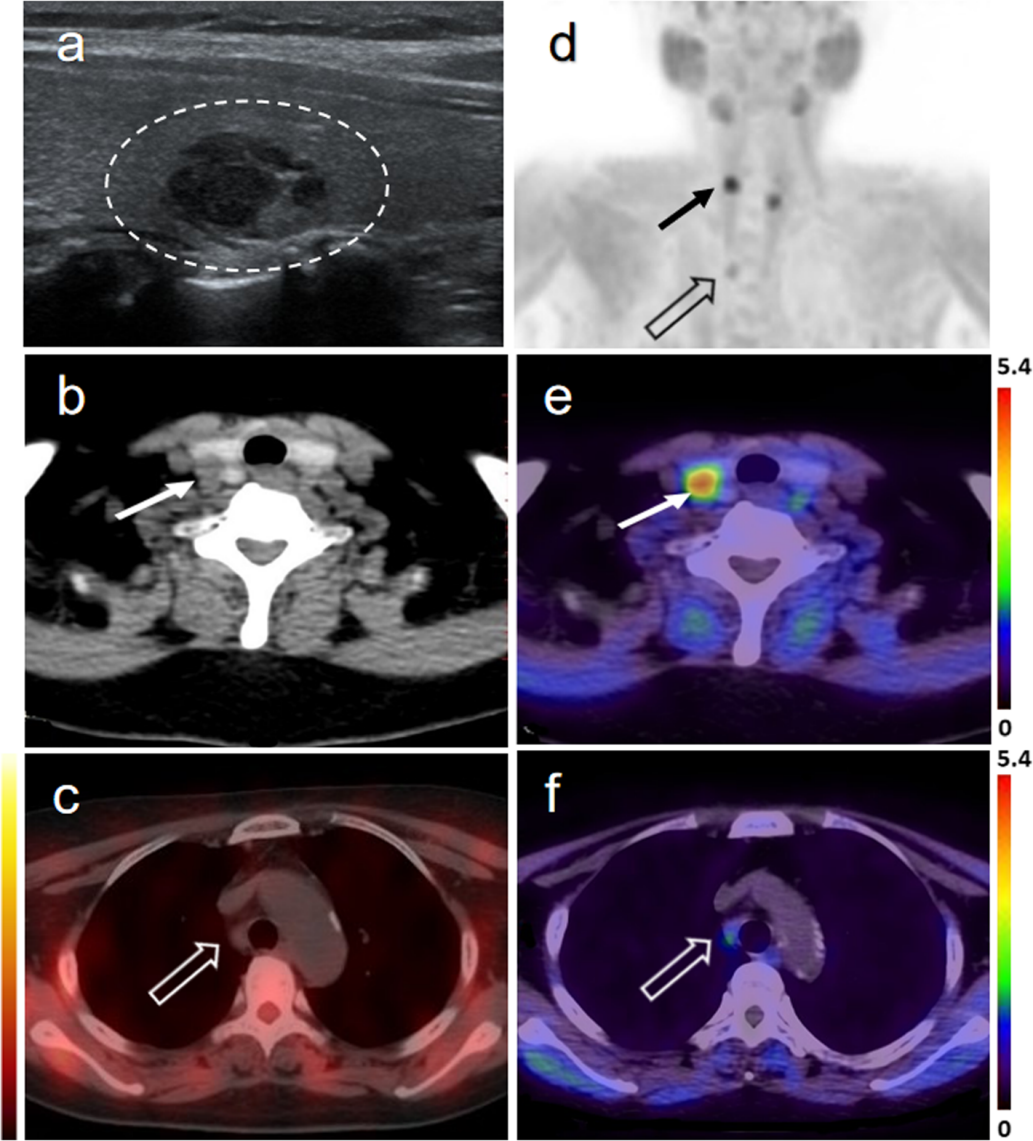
Images from a 61-year-old woman with multiple parathyroid hyperplasia, one with a slightly ectopic (in the middle portion of the right thyroid gland) localization. US misdiagnosed this lesion as a thyroid nodule (dotted circle) (a). The lesion is well delineated on the MIP image of ^18^F-FCH PET (d), axial CT (b), and PET/CT fusion images (e) (arrows). A heterogeneous low-density nodule in the upper mediastinum is well-delineated on the MIP image of ^18^F-FCH PET (d) and PET/CT fusion images (f) (hollow arrows), but no abnormal tracer uptake was observed on a ^99m^Tc-sestaMIBI SPECT/CT image (c) (hollow arrow)
